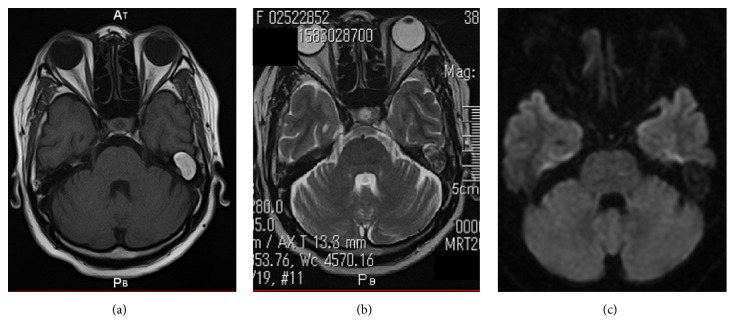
(a) Axial T1-weighted temporal bone MRI showing a signal-hyperintense mass neighboring the posterior cranial fossa and extending to the middle fossa. (b) Axial T2-weighted imaging of the temporal bone shows a high-intensity periphery and low-intensity central mass. (c) DWI shows an isointense signal rather than high intensity.
